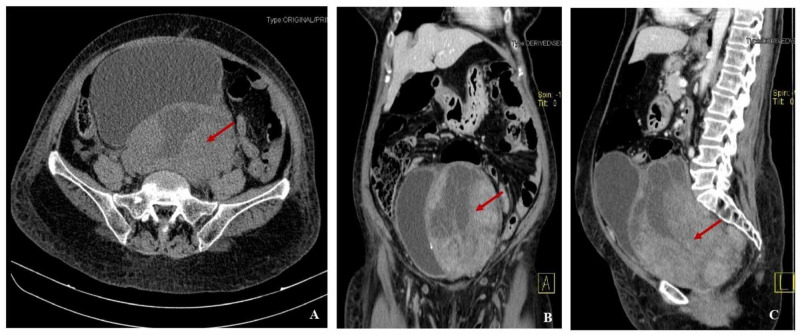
Contrast-enhanced CT image showing a pelvic large mass, measuring 17 cm of greater diameter, heterogeneous, well-defined, with cystic areas, deviating the bladder (arrows) (A) Axial section; (B) Coronal section; (C) Sagittal section CT: computed tomography
